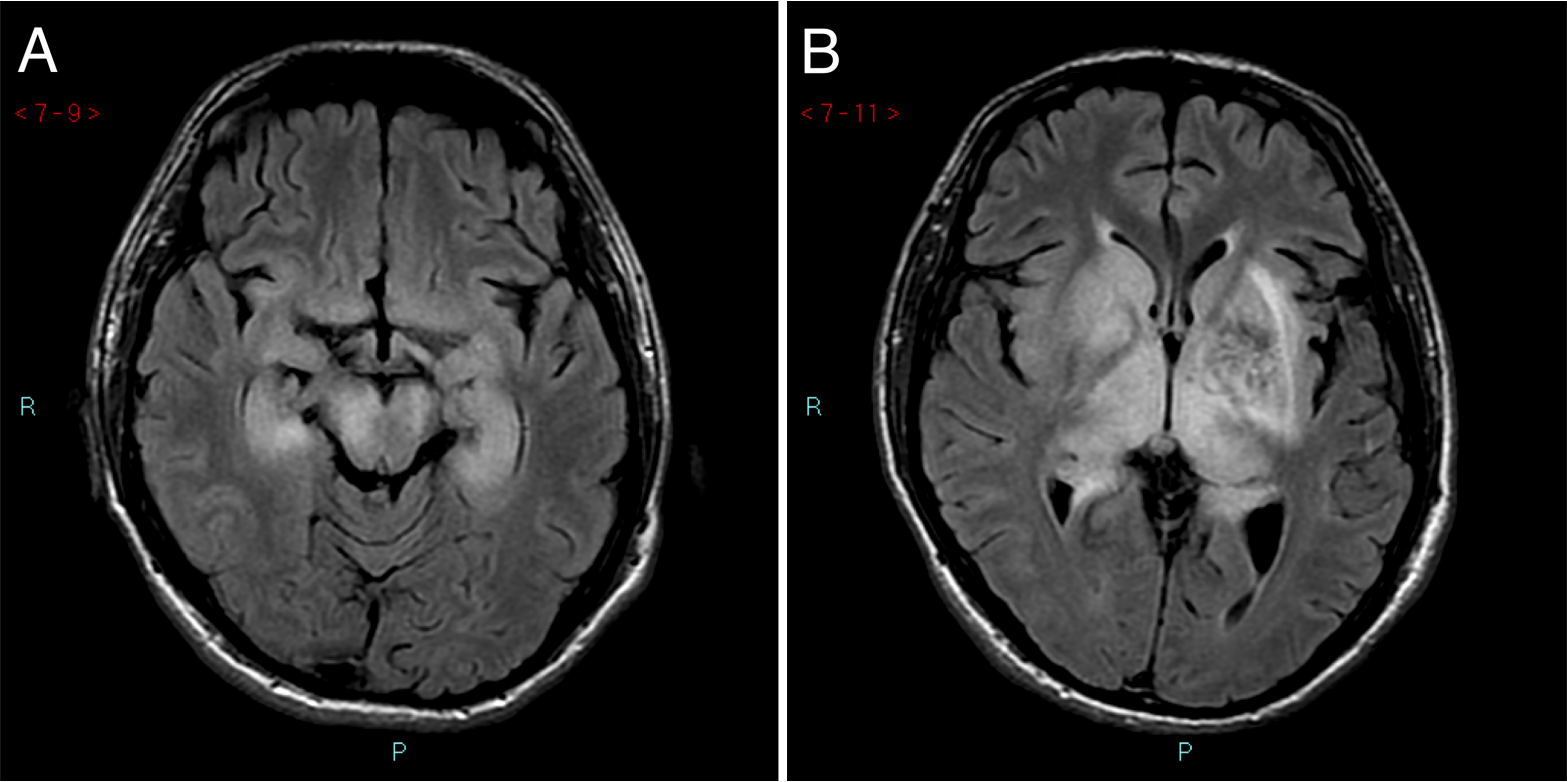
MRI of Patient three. (A), FLAIR images from an MRI scan performed in Bangkok on 5 August 2015 showing symmetrical high signal affecting the thalami and lentiform nuclei bilaterally. Evidence of radiosurgery to the left basal ganglia arteriovenous malformation with associated encephalomalacia within the left cerebral white matter and some haemosiderin deposition from the arteriovenous malformation is seen. (B), The abnormal area extends into the medial temporal lobes, the cerebral peduncles and into the brain stem
